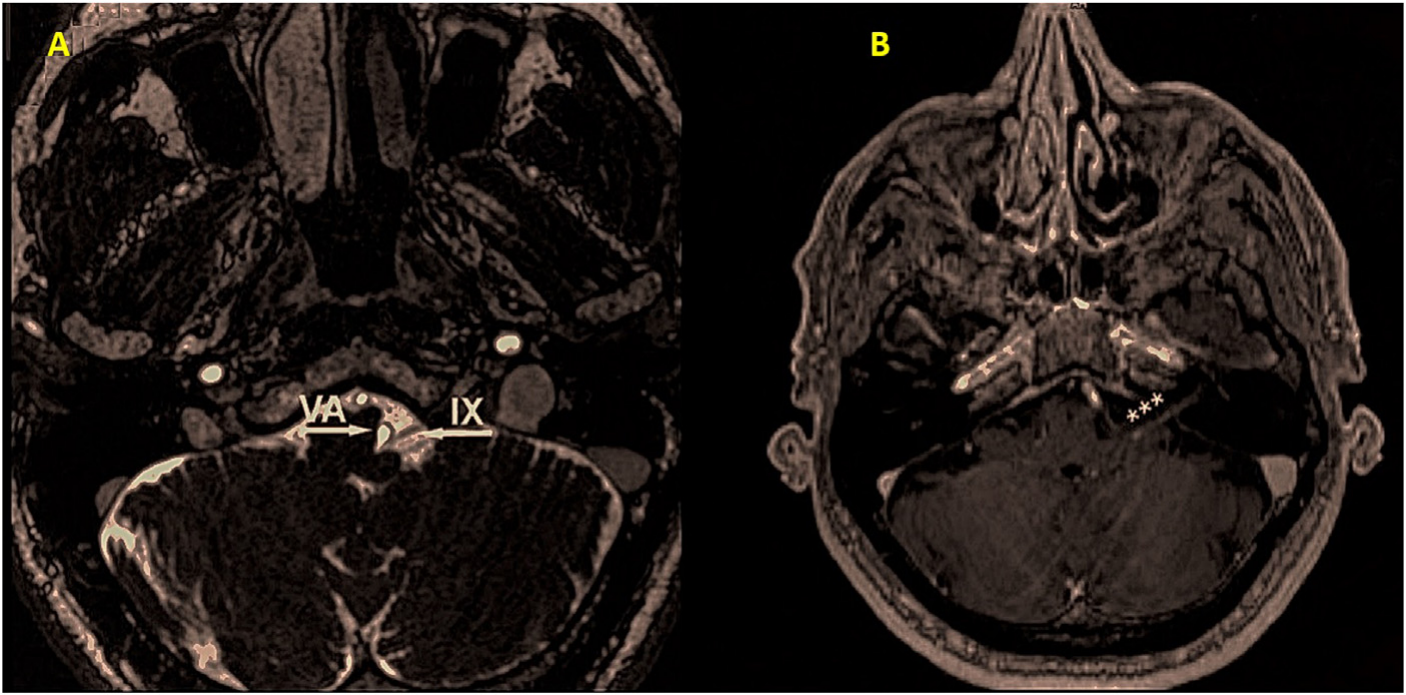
(A) T2-weighted and (B) T1-weighted contrast-enhanced images of left glossopharyngeal (IX) nerve after gadolinium, confirming neurovascular conflict with the vertebral artery (VA), which seems to compress the proximal portion of the nerve.
